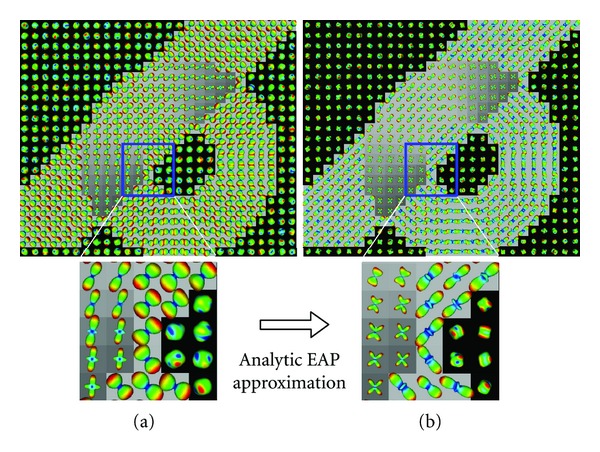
Synthetic dataset experiment. Two fiber bundles intersecting with the DWI signal corrupted by a Rician noise of SNR = 30. (a) $\hat{\text{ADC}}$ from 4th-order tensors. (b) Analytically approximated EAP, Pr_4,4_
